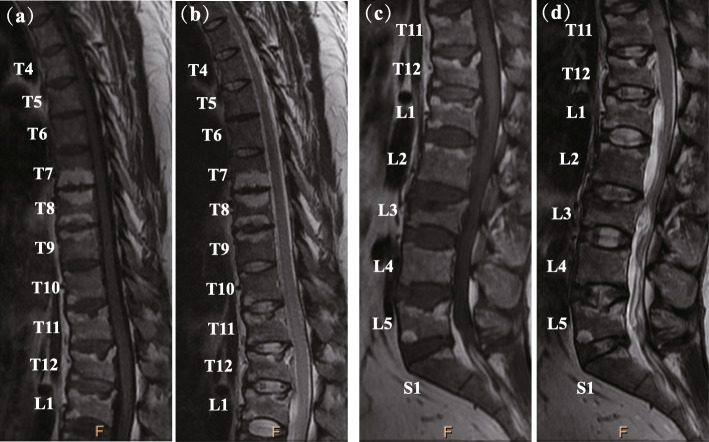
Magnetic resonance imaging (MRI) of the thoracolumbar spine. L3 vertebral compression fracture, local kyphosis, spinal canal stenosis, and cauda equina compression were observed. Vertebral endplate alterations were found in L1 to S1, and L2-S1 disc bulges and slight L4-S1 disc protrusions were noted. Schmorl’s nodes were observed in multiple thoracic and lumbar vertebrae (T8, T12, L1, and L5). (a) T1WI of the thoracic vertebra. (b) T2WI of the thoracic vertebra. (c) T1WI of the lumbar vertebra. (d) T2WI of the thoracic vertebra. T1WI, T1-weighted images; T2WI, T2-weighted images
